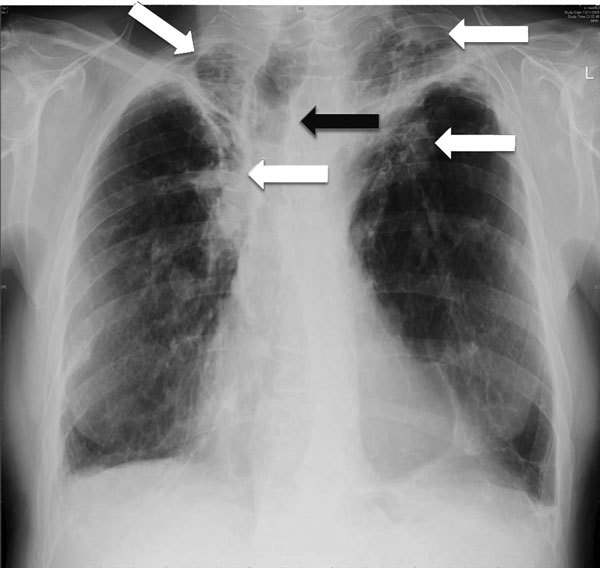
Chest radiograph showing bilateral upper lobe chronic pulmonary aspergillosis, which can be easily mistaken for pulmonary tuberculosis. White arrows indicate areas of abnormality (some pleural thickening and opacification) in both apices, which are similar, although slightly more obvious, to findings in pulmonary tuberculosis. Black arrow indicates the trachea pulled to one side by the contraction and fibrosis on that side. Image used with permission of David Denning (©2016, all rights reserved).
